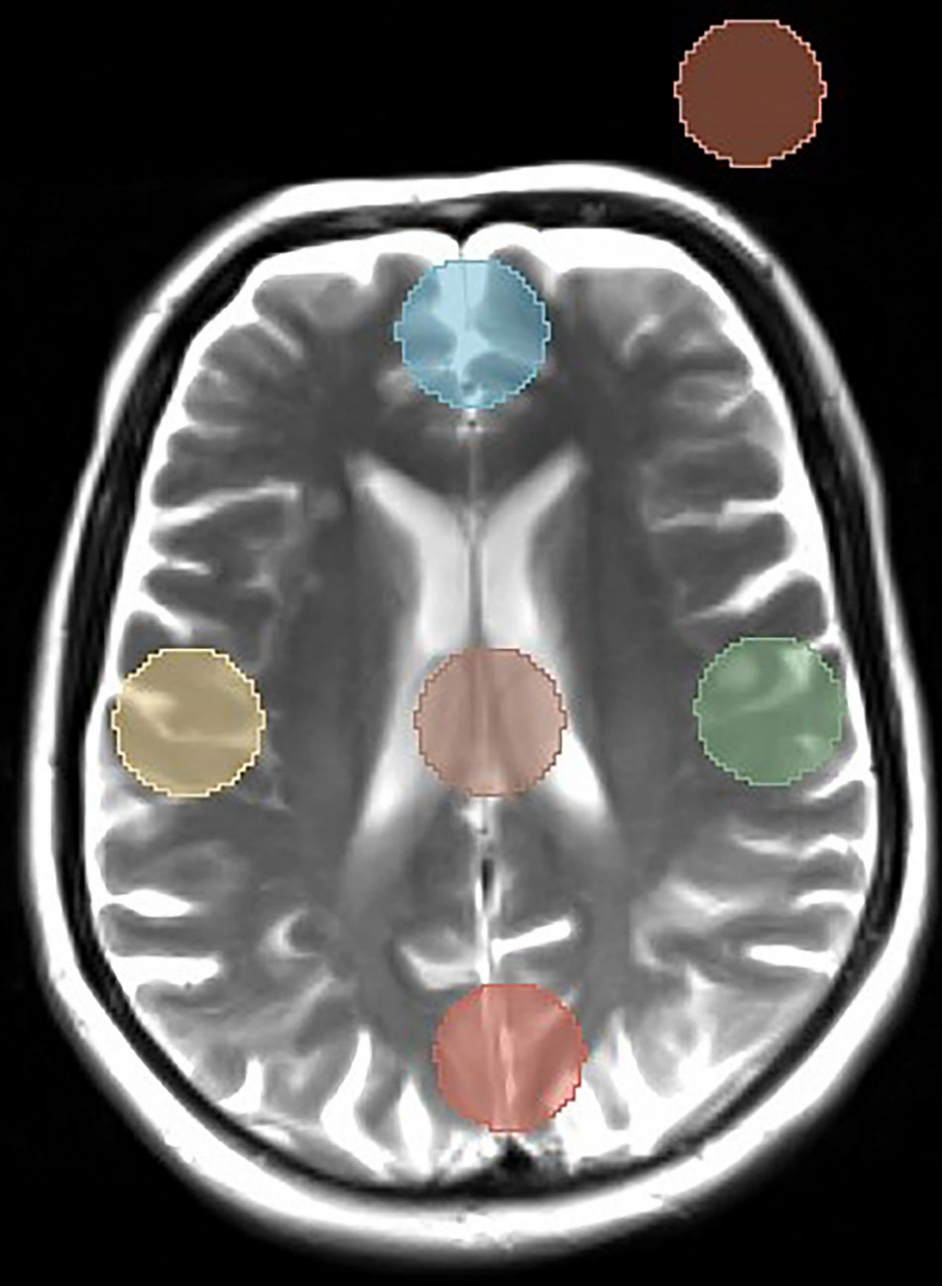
Delineation of six ROIs for SNR calculation of MRI images in RT and DS setups.
